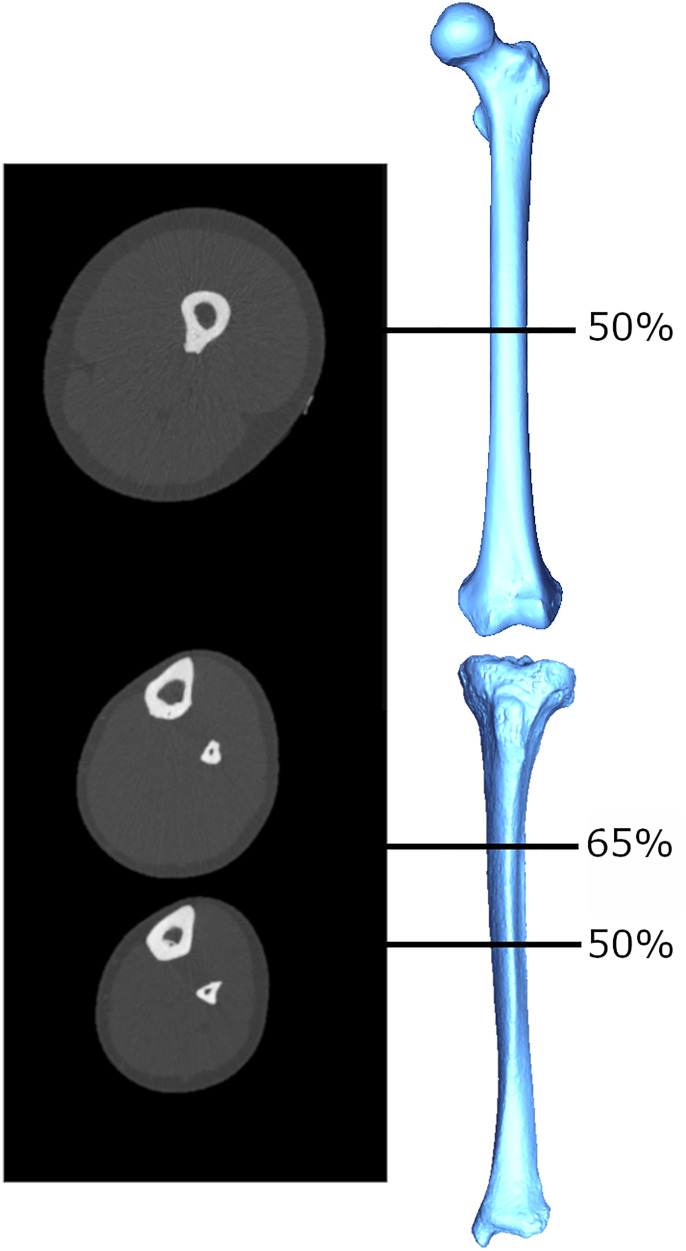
Example cross-sectional images obtained using peripheral quantitative computed tomography (pQCT), from which limb bone CSG properties and MCSAs are quantified. The mid-thigh image is taken at 50% of femoral length, and the lower leg images are taken at 65% and 50% of tibial length. Greyscale coloration represents tissue density: white (most dense) = bone, light grey = lean mass, dark grey (least dense) = fat mass.
